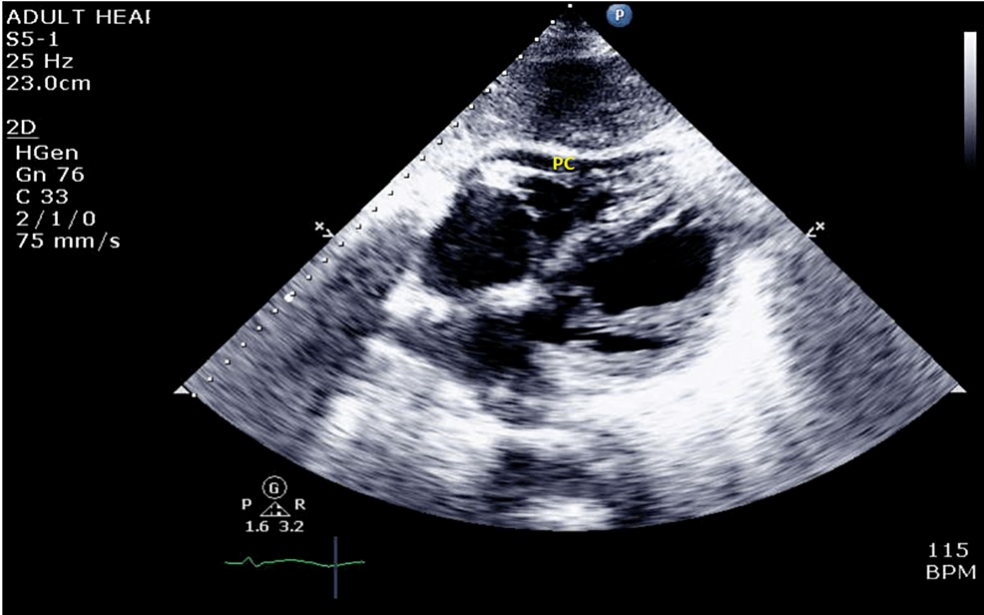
Pericardial effusion on echocardiography. PC: pericardium
